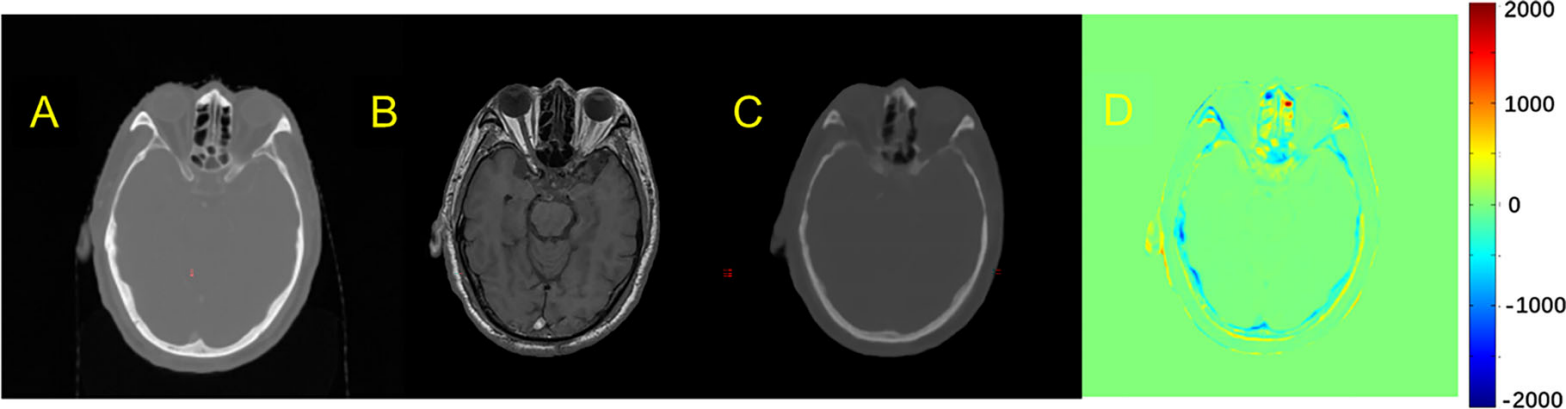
Comparison example of pCT and original CT: (A) Original CT images; (B) T1-weighted MR images; (C) Predicted pCT images; (D) Difference between the real CT and predicted pCT values, where MAE is 73.1 HU.
